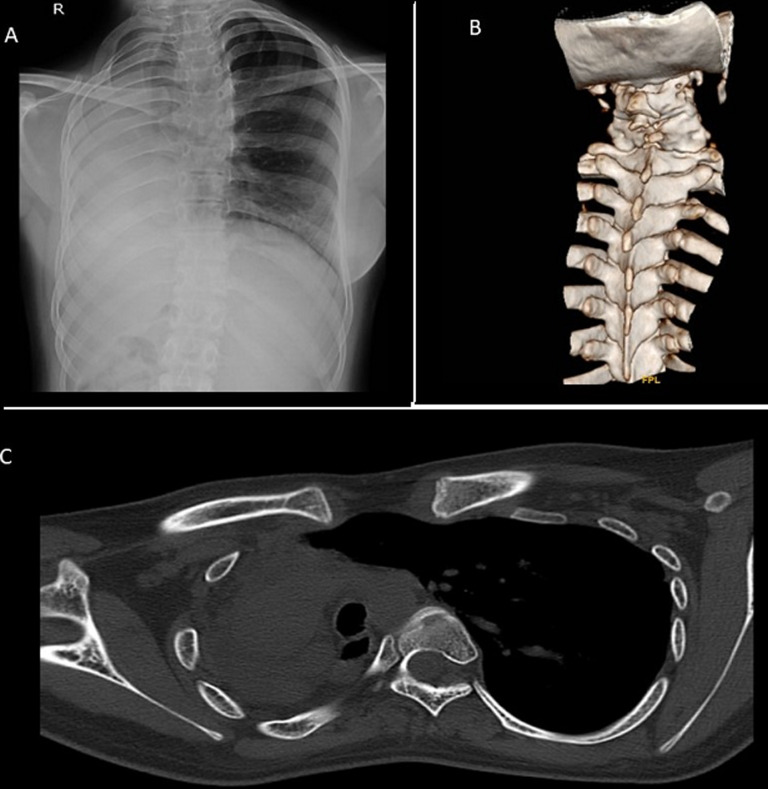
A) chest X-ray of the patient showing right opaque hemithorax with ipsilateral mediastinal shift; B) 3D reconstruction of the vertebra showing fusion of cervical vertebra suggesting segmental anomaly; C) computed tomography of thorax showing underdevelopment of the right lung with mediastinal shift to the right with compensatory hyperinflation and herniation of the left lung
